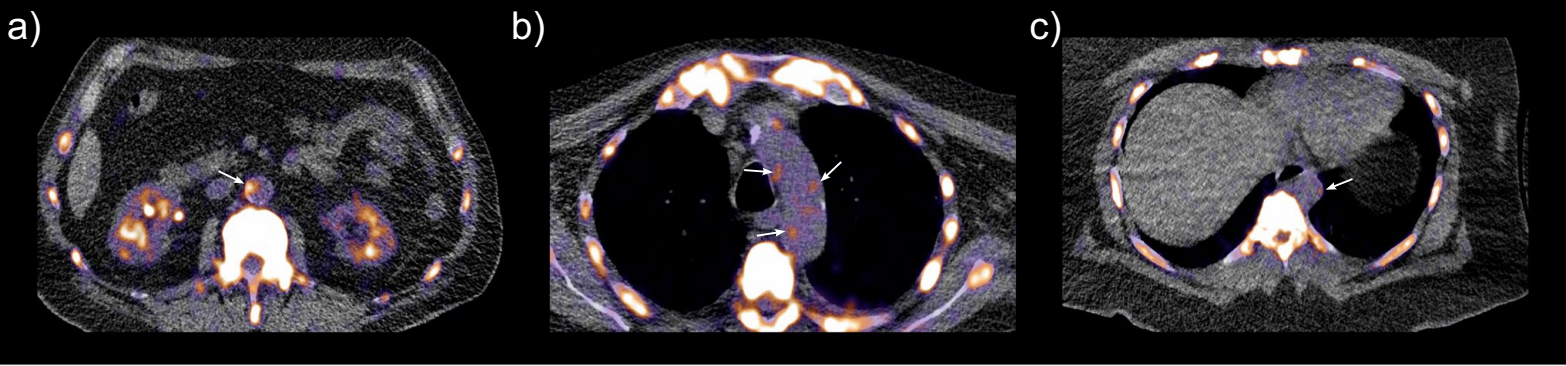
Examples of Na[^18^F]F uptake on PET and related to macrocalcifications on CT. a Na[^18^F]F uptake adjacent to a macrocalcification on CT; b Na[^18^F]F hotspots in the vascular wall of the aortic arch, but not near any macrocalcifications; c a patient with no calcifications, but some Na[^18^F]F hotspots in the vascular wall
